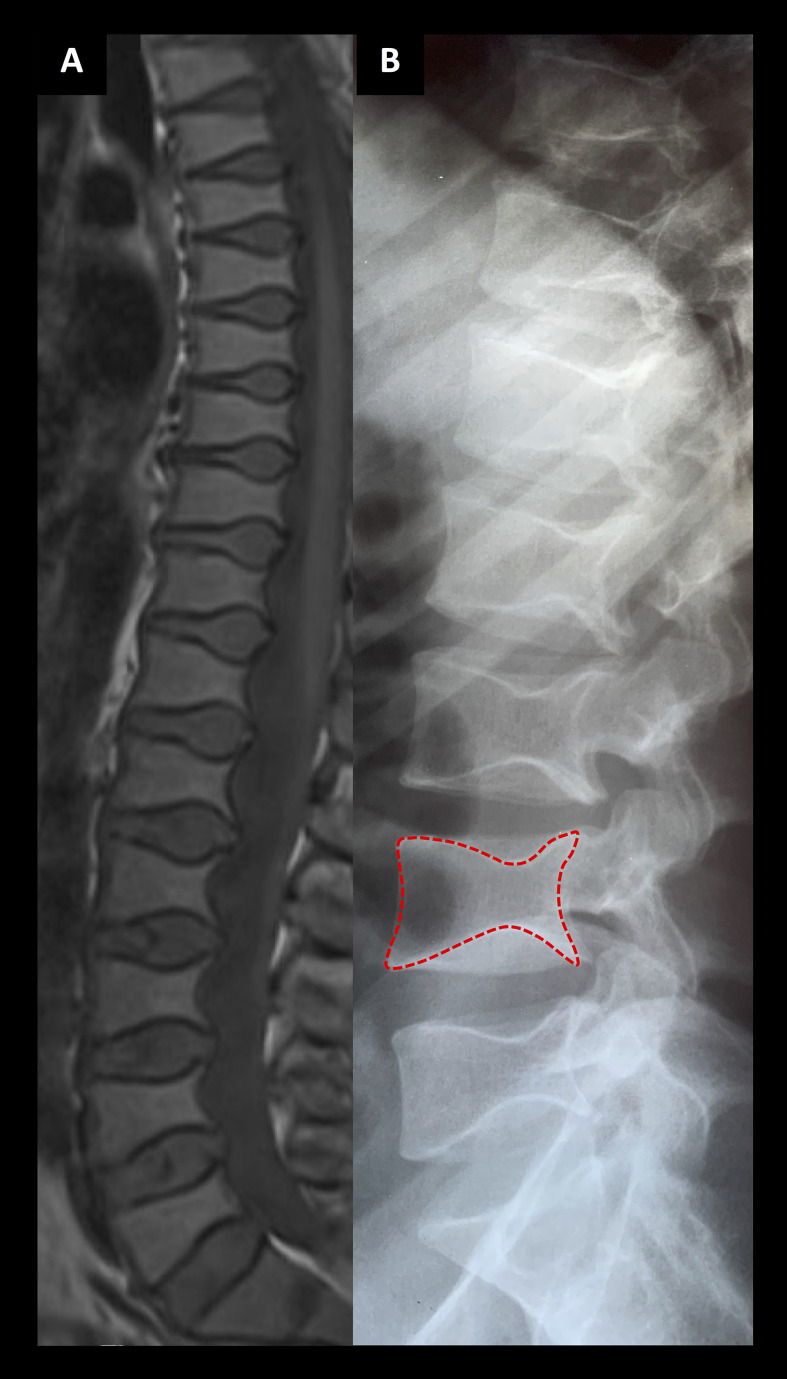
Spinal images of two patients associated with a novel *TBX2* missense variant p.Thr300Met. (A) An MRI section of the female index at age 24 years and (B) a lumbar radiograph of her father at age 24 years showing codfish-type vertebral dysplasia with balloon-shaped intervertebral discs.
